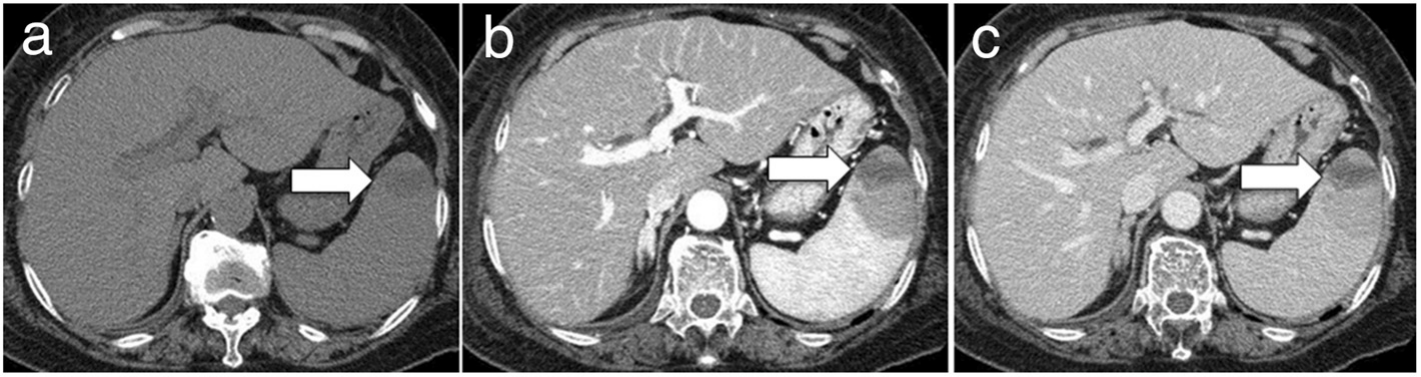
Contrast-enhanced abdominal computed tomography image of the abdomen. (a) Plain phase. (b) Arterial phase. (c) Equilibrium phase. Splenomegaly and multiple spleen tumors were observed. The maximal tumor size was 52 mm and the tumor had a necrotic lesion (white arrows).
